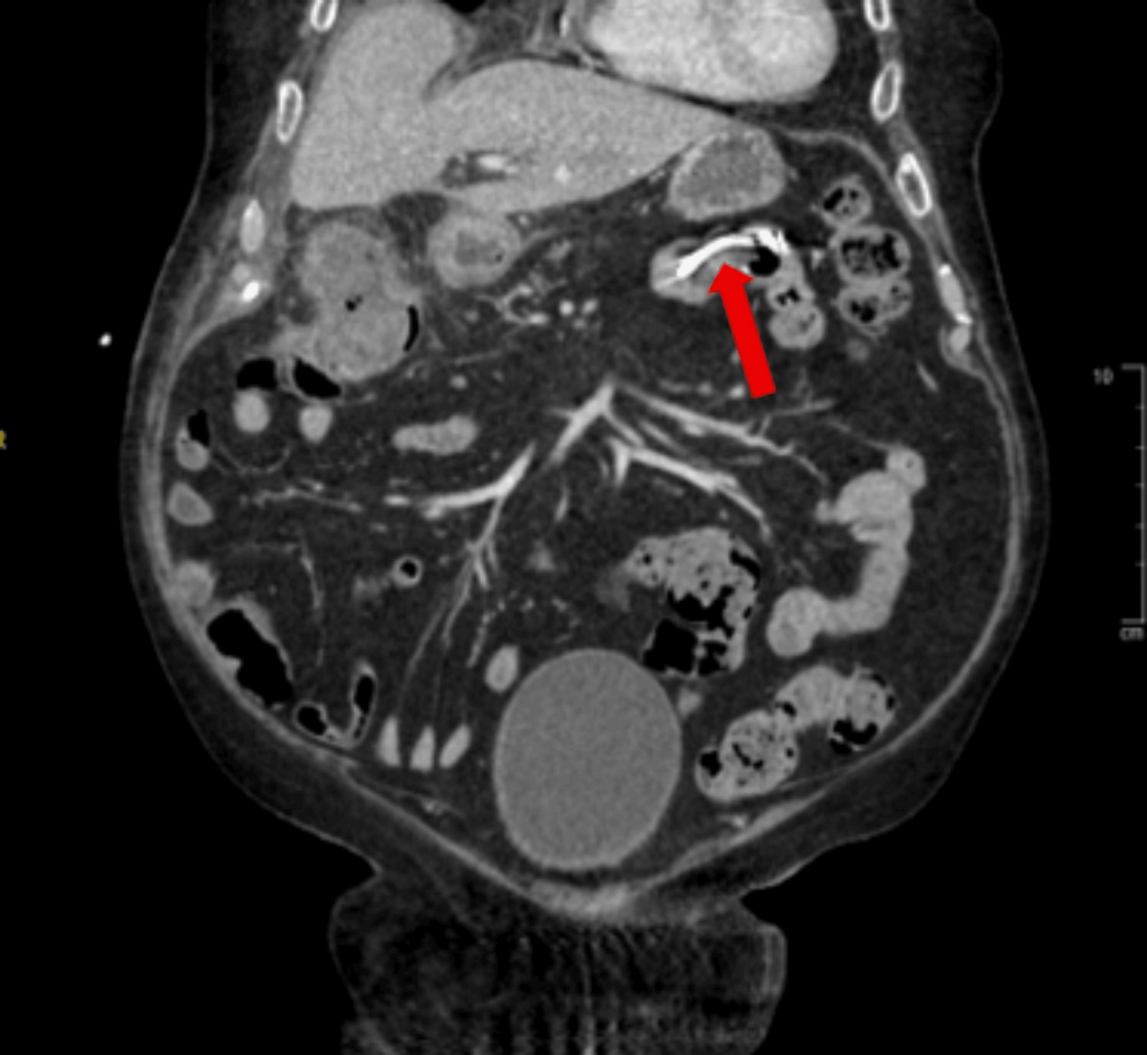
Coronal CT showing common bile duct stent (red arrow) visualized in the distal duodenum and proximal jejunum, indicative of migration.
